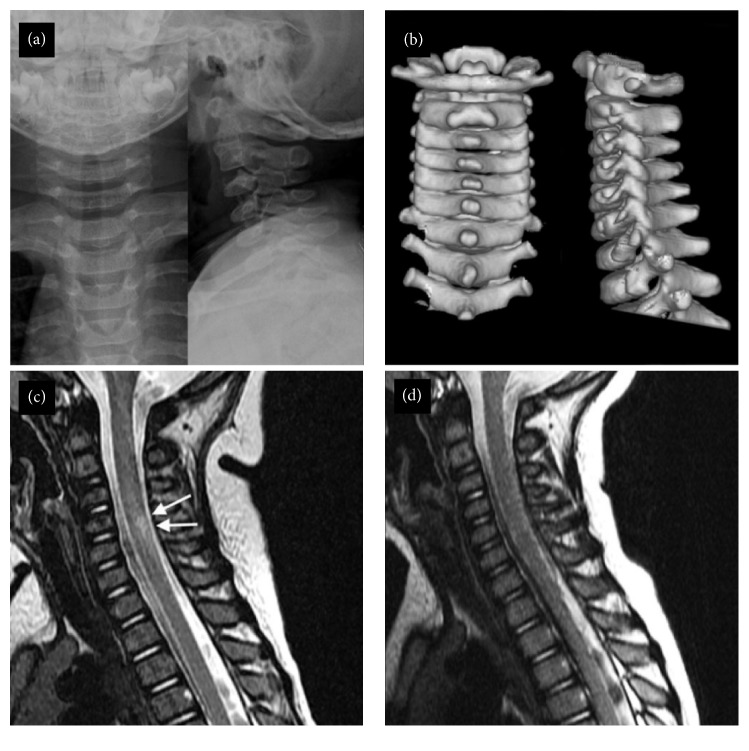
A 12-month-old female infant presented with nausea, vomiting, and drowsiness to emergency room after falling from a height of less than 30 cm. She had no neurological deficit at presentation, and cervical spine plain radiographs (a) and CT with 3D reconstruction (b) showed no abnormal findings. (c) Seven days after the injury the patient developed right sided hemiparesis and cervical MRI revealed increased intensity (arrows) in the T2-weighted images at the level of C6. (d) Repeat cervical MRI one month later shows that increased signal intensity has disappeared. The patient continued to improve neurologically until 24 months after the injury and returned to near-normal.* (Source: with permission from [17].)*
